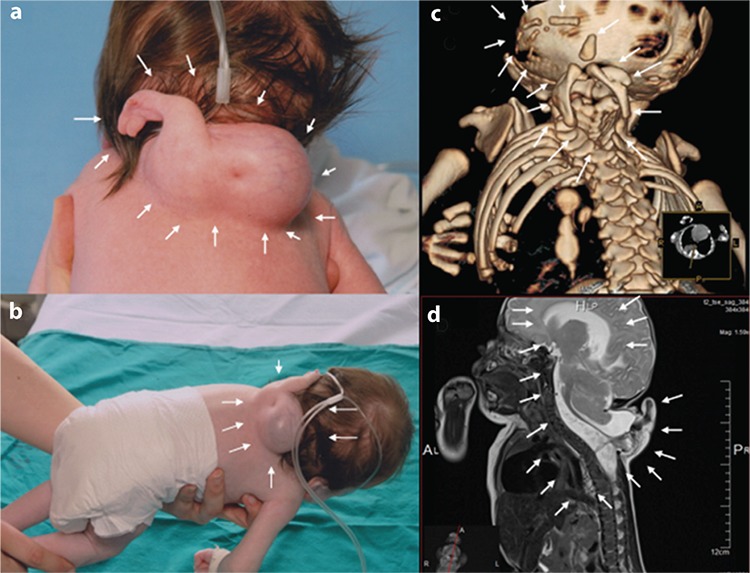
a-d. Meningomyelocele of the autosite and the rudimentary accessory upper limbs of the “parasitic” twin attached to the autosite (a, b), 3D-computed tomography revealing the fusion location of the accessory limbs of the “parasitic” twin with the autosite. There are two hypoplastic scapulae of the parasitic twin behind Th7-9, one hypoplastic humerus and ulna, one hypoplastic carpal bone, and five hypoplastic phalangeal bones of the two digits. In complete fusion of the posterior arches of the cervical and thoracic vertebrae (c), magnetic resonance imaging revealing eccentric meningomyelocele, ventriculomegaly, simplified gyral patterning, the deformation of the spinal cord along C1-Th12, and a Bochdalek hernia (d).
